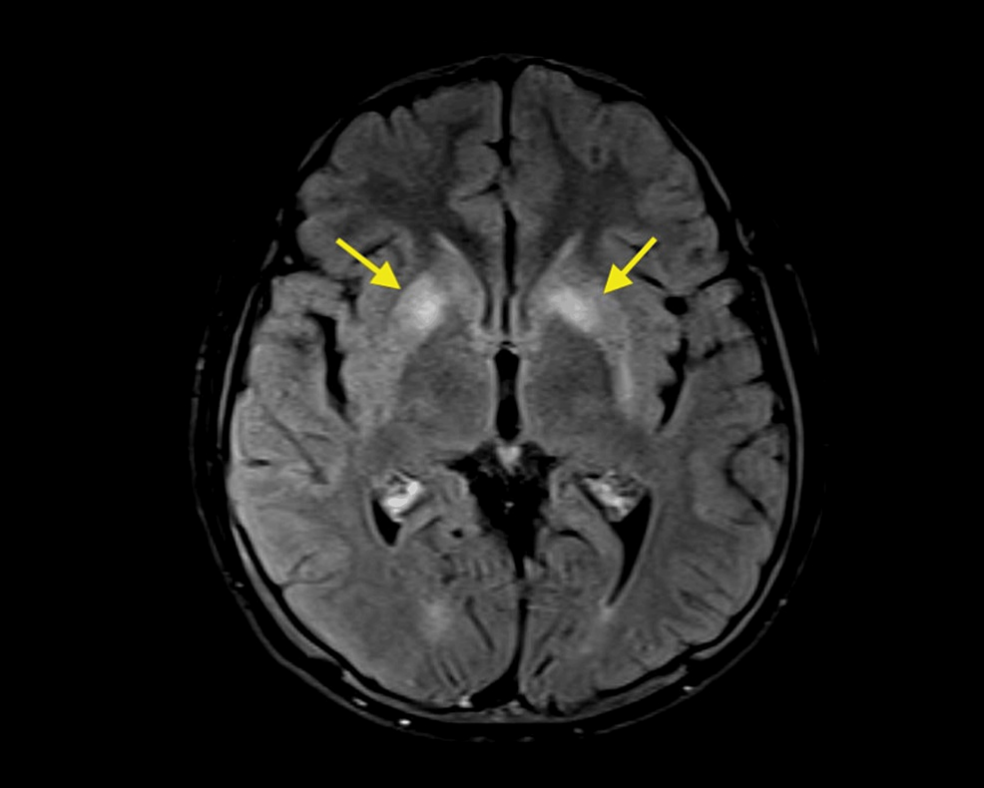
MRI STIR transverse section of the brain Yellow block arrows show hyperintensity in the bilateral ganglio-capsular region MRI: Magnetic resonance imaging, STIR: Short tau inversion recovery
